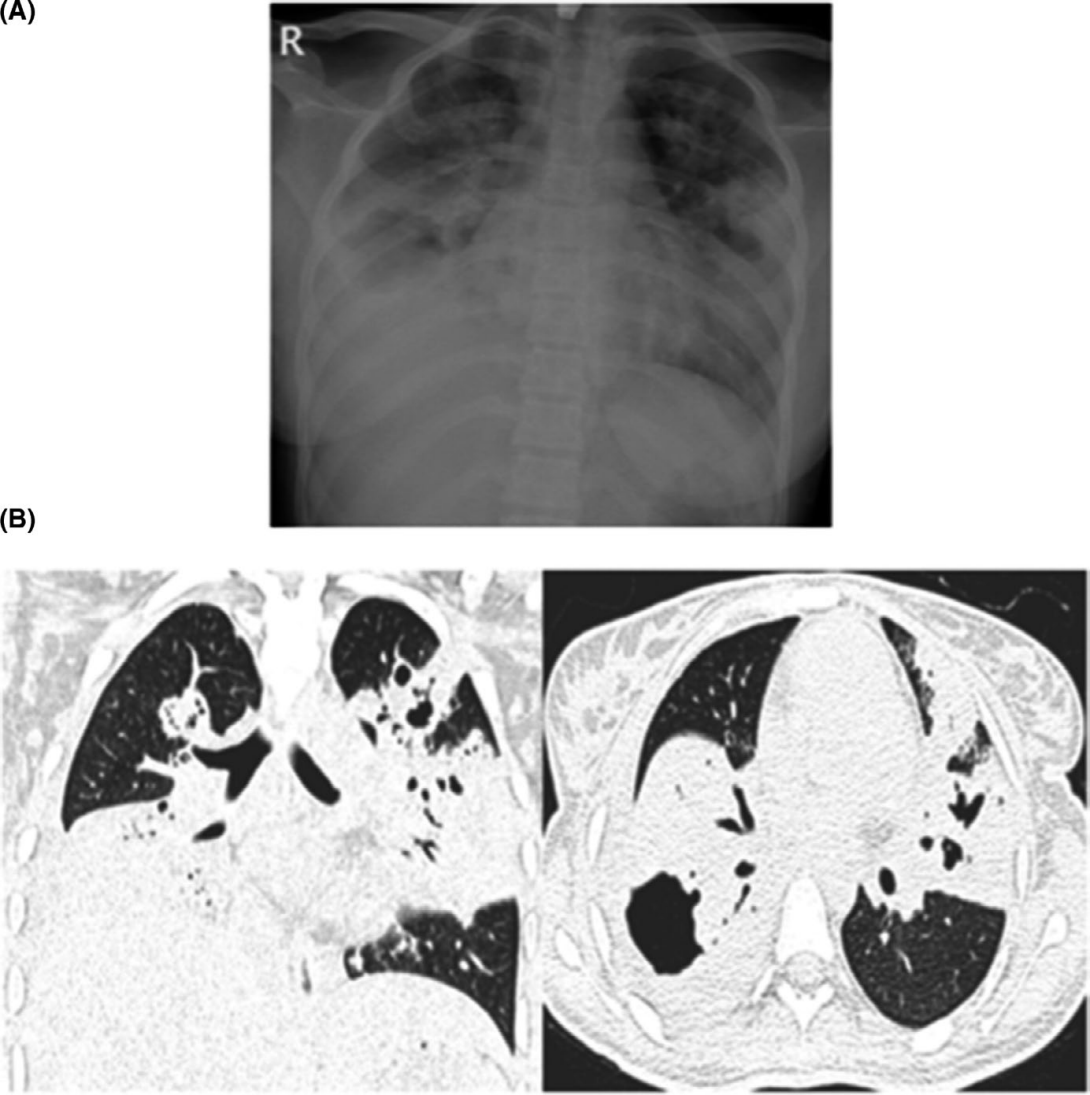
A, CXR showed bilateral patchy consolidation. B: CT chest images showing bilateral patchy consolidation, multifocal broncheactasis, unilateral pleural effusion, and calcified mediastinal lymphadenopathy
